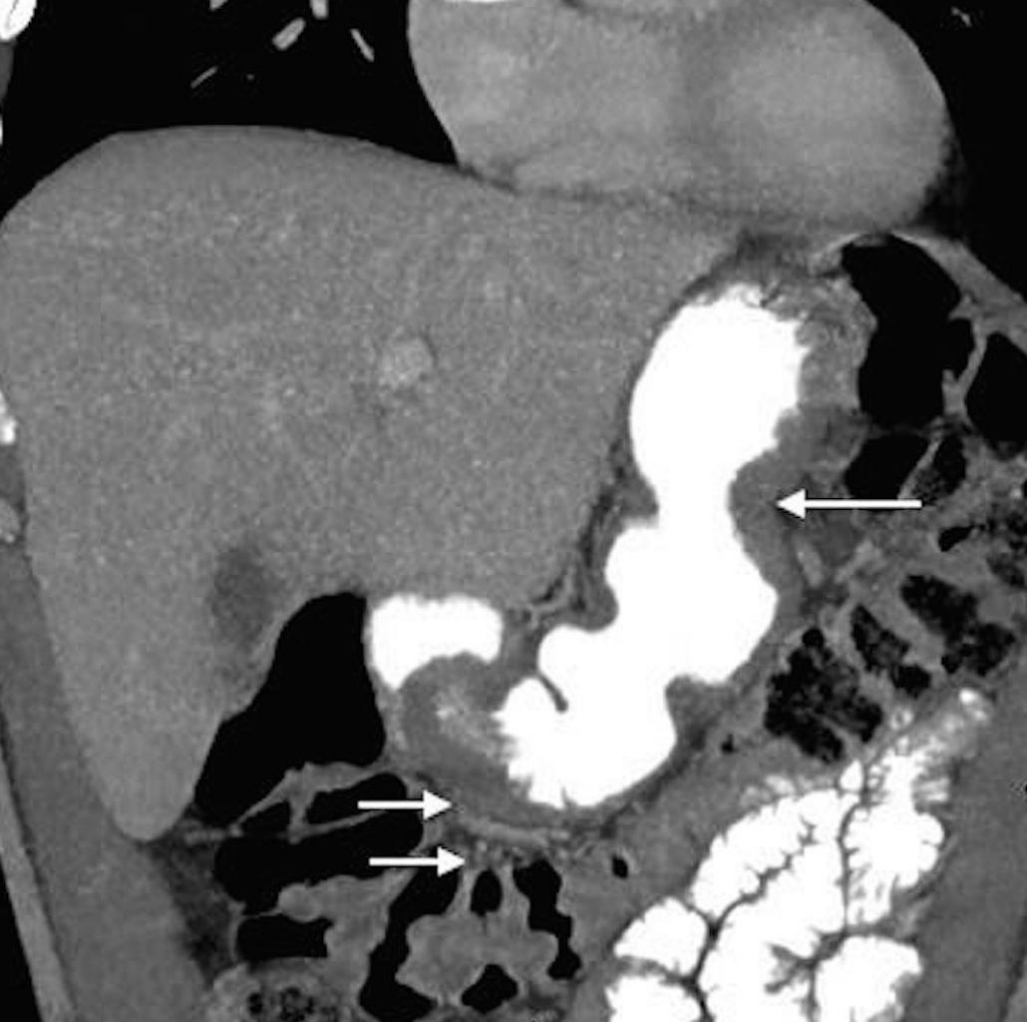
Coronal oblique 5 mm MIP reconstruction CT image taken from Patient 1 after four cycles of FOLFOX. This image shows significant interval decrease in disease burden with a marked decrease in the size of the primary gastric mass in addition to a complete resolution of the previously documented infiltration of adjacent fat and extensive mesenteric lymphadenopathy.
